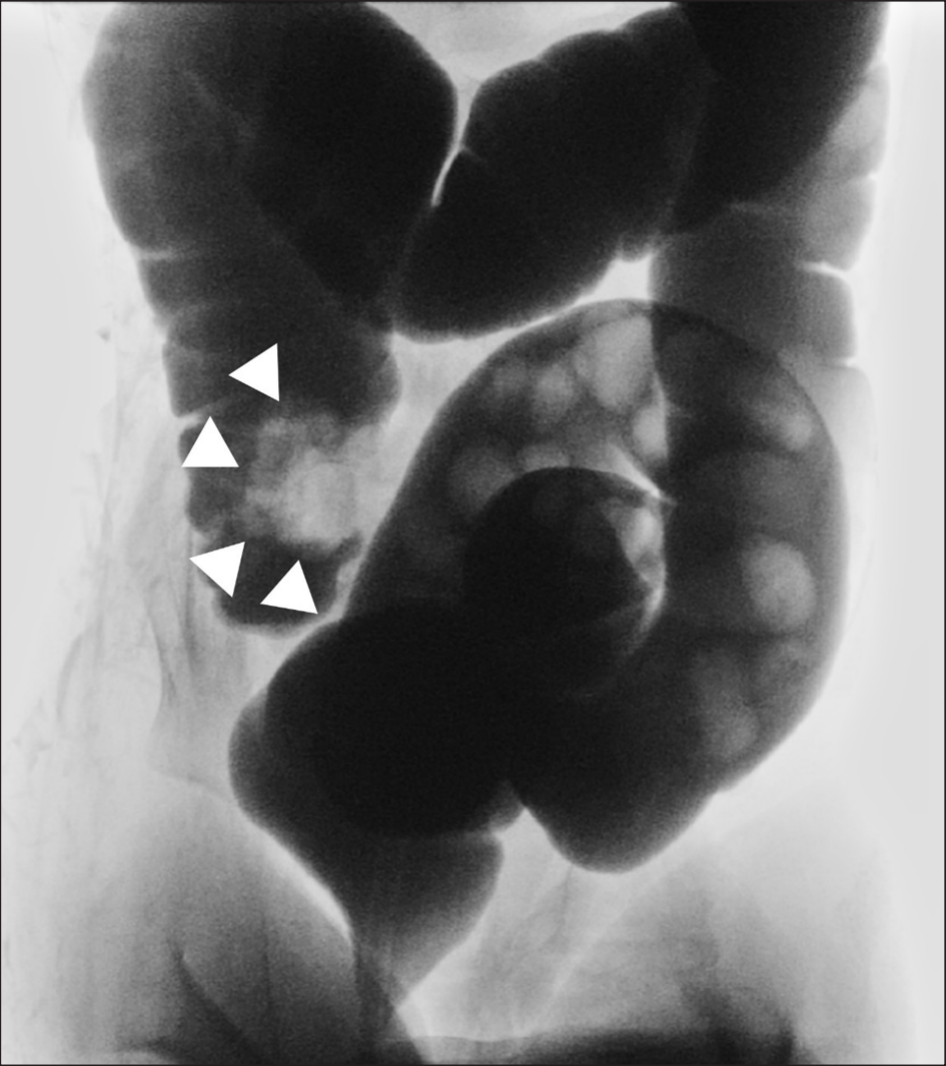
Abdominal ultrasound showed a small target sign in the transverse plane without presence of mesenterial fat, vessels or lymph nodes between the layers of the target sign (Figure 2). The width of the presumed intussusception was 25 mm over a short segment of 16 mm. The cecum was oriented as normal, and the appendix was in a normal position. In retrospect this correlated with the external single-contrast enema images (Figure 1). The patient had a second episode of ileocolic invagination within 12 hours, which was again reduced with hydrostatic reduction under fluoroscopy (Figure 3). After this second reduction, he was administered for observation and normal intake was resumed the next day, without recurrence. Follow-up ultrasound 48 hours after the first episode demonstrated an important reduction in ileocecal valve size and the patient was discharged after 48 hours.
